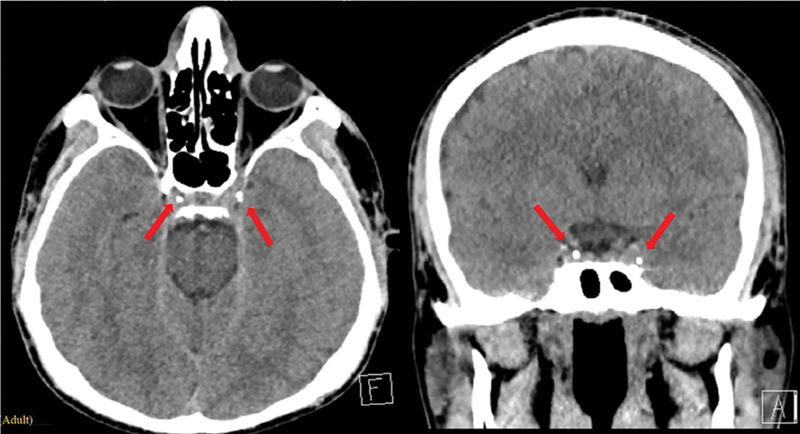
CT-scan images demonstrating glue migration into the bilateral cavernous sinuses. Glue emboli are indicated by red arrows in the images.
